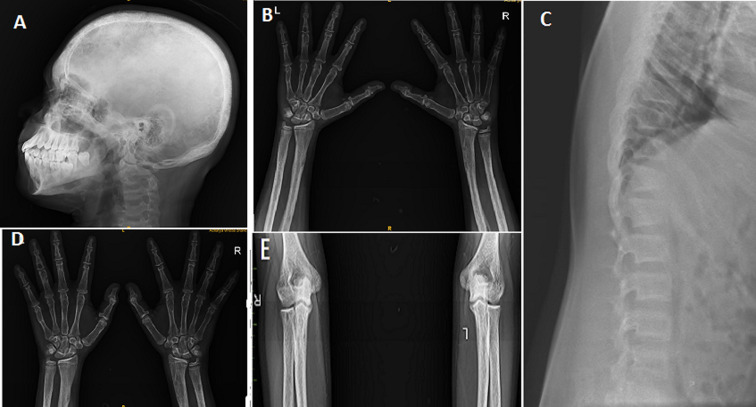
A) X-ray of thinning and increased translucency of the cranial bones, particularly in the occipital area of skull; B) X-ray of widened growth plate, metaphyseal fraying, splaying and cupping of the distal radius and ulna seen; C) X-ray of Rachitic rosary (beaded appearance on X-rays); D) X-ray showing cupping and fraying of the metaphysis of distal end of radius; E) X-ray of cupping and metaphyseal fraying and splaying of the distal humerus and proximal radius and ulna
